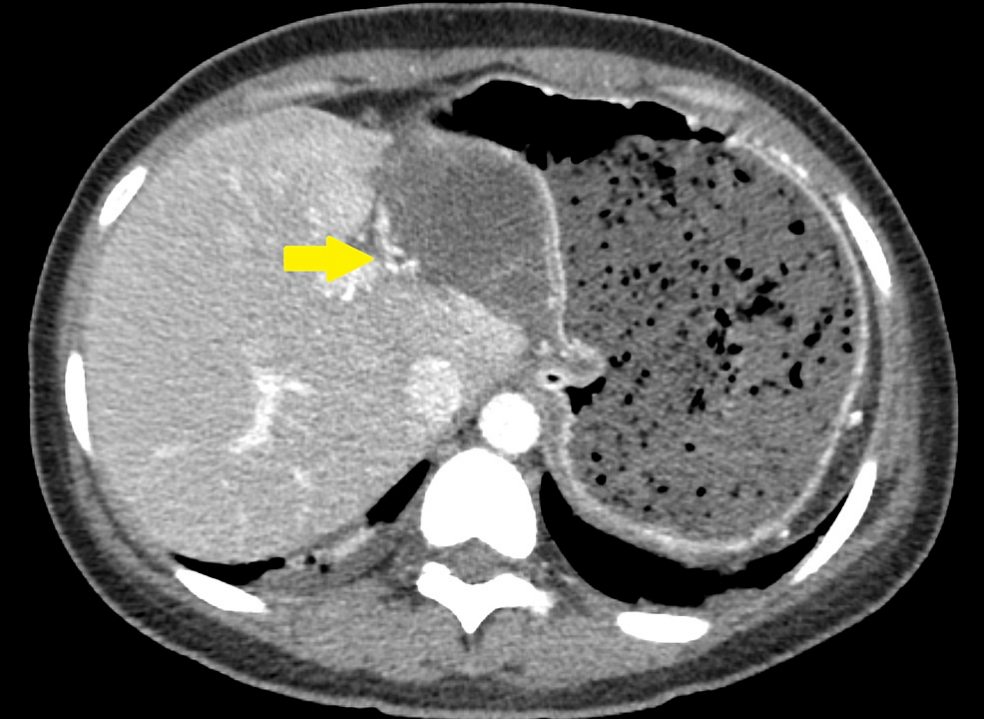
Axial CT scan in arterial phase shows twisted gallbladder vascular pedicle (arrow)
